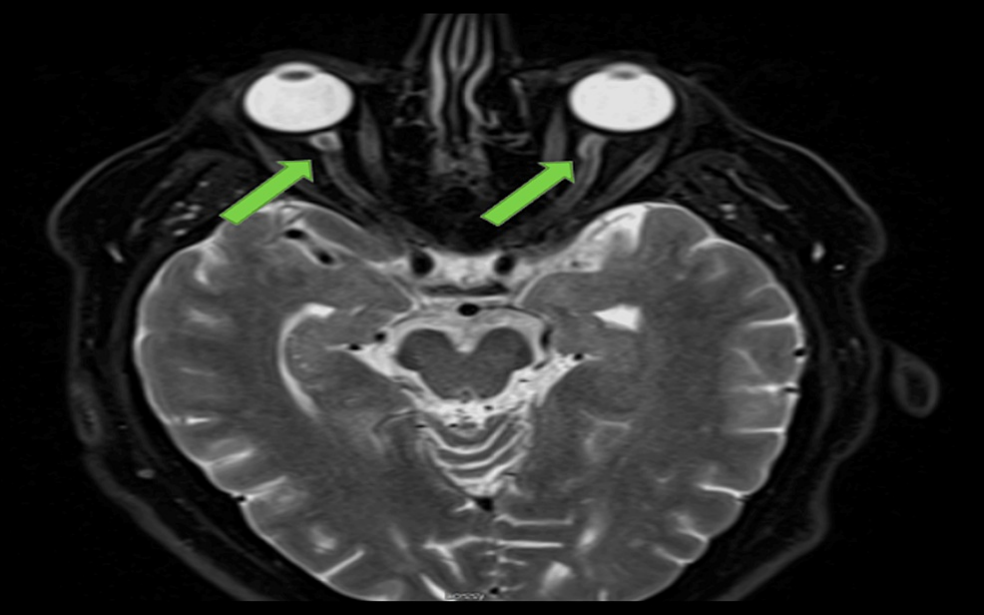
MRI brain/orbit showing minimal to mild wavy contour noted to the optic nerve with tram track appearance, green arrows on T2-weighted imaging suggestive of mild optic nerve edema, left greater than right
